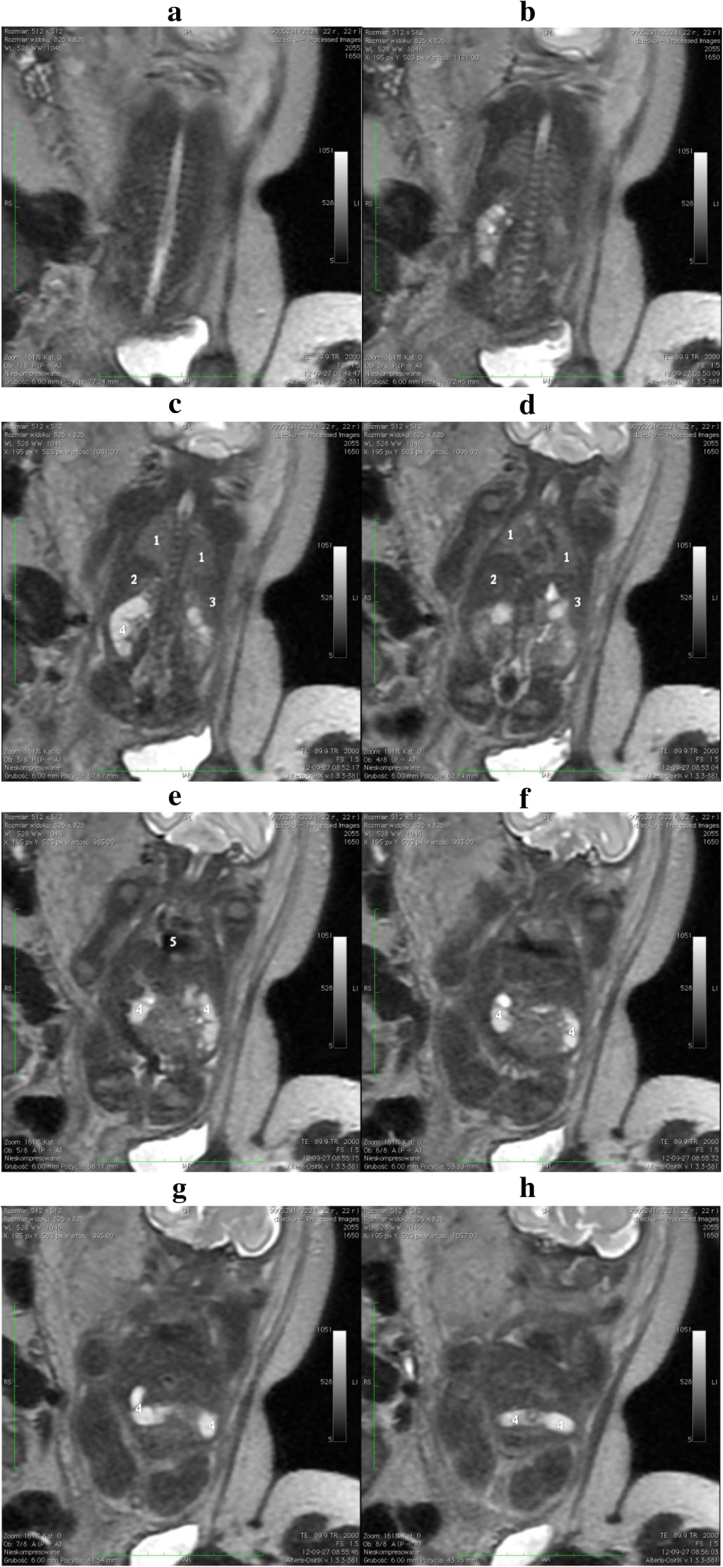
Images of the coronal plane from the dorsal to ventral side of the fetus (a-h). Single-shot fast spin-echo sequences, T2-weighted images. 1, lung; 2, liver; 3, spleen; 4, intestine; 5, heart.
